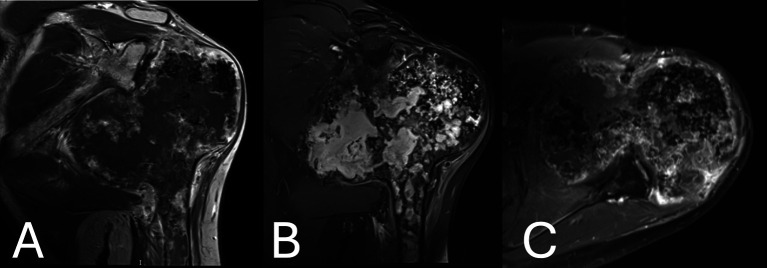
(A) Magnetic resonance imaging showing the exuberant medial compartment, denoted as a large lobulated area, extending to the cortex, with low signal intensity on T1W. (B) Magnetic resonance imaging showing High signal intensity on fat-suppressed T2 W images of the lobulated area on the MRI. Flocculent stippled areas of a signal void, representing cartilaginous calcifications, were more prominent in the lateral compartment. Geographic areas of marrow signal, which are high on T1W and low on fat-suppressed T2W, were entrapped within the cartilaginous tissue of the medial compartment, whereas bone marrow signal was seen at the periphery of the lateral compartment. Cortical disruption was seen at the posterior aspect of the epiphysis corresponding to the osteolytic areas shown on CT. (C) Magnetic resonance imaging post gadolinium administration showing mild peripheral inhomogeneous enhancement. Tissue enhancement was more diffuse or nodular and somewhat more intense at the posterior aspect adjacent to the osteolytic area. Lobules of cartilaginous signal intensity were also seen in the middle and lateral components of the epiphysis, extending to the metaphysis.
